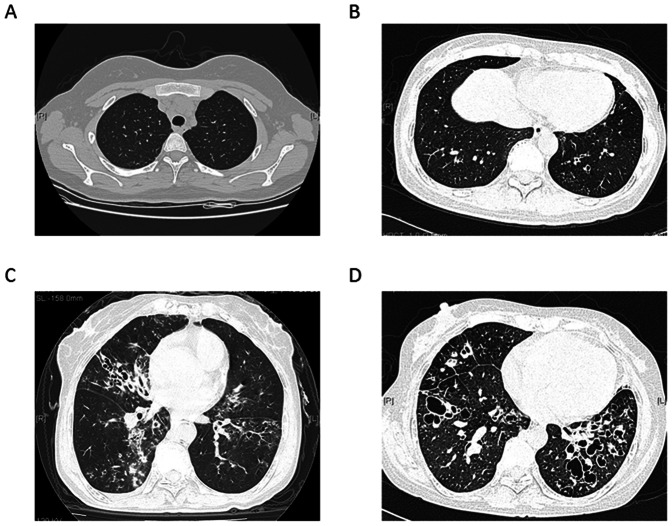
HRCT grading system for patients with bronchiectasis. Each lobe of both lungs is graded for bronchiectatic changes on a scale of 0 to 3 (lingula was scored as a separate lobe), giving a maximum of 18 points. (A) Represents score 0: no bronchiectasis. (B) Represents score 1 in one lobe: one bronchopulmonary segment involved (C) Represents score 2 in one lobe: more than one bronchopulmonary segment involved. Total Score was 8 in this figure due to four lobes involved. (D) Represents score 3 in one lobe: grossly cystic bronchiectasis. Total Score was 12 in this figure due to three lobes involved.
